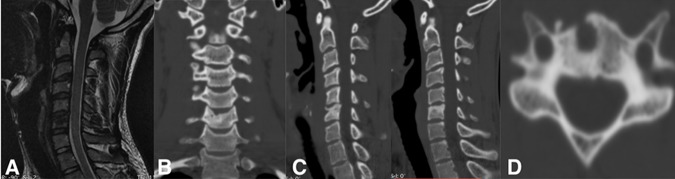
One-year follow-up imaging depicting (a) MRI sagittal view with preservation of adjacent disc; (b) CT scan coronal; (c) sagittal; and (d) axial views with filling of cavity with new bone formation and preservation of disc height and stability.
